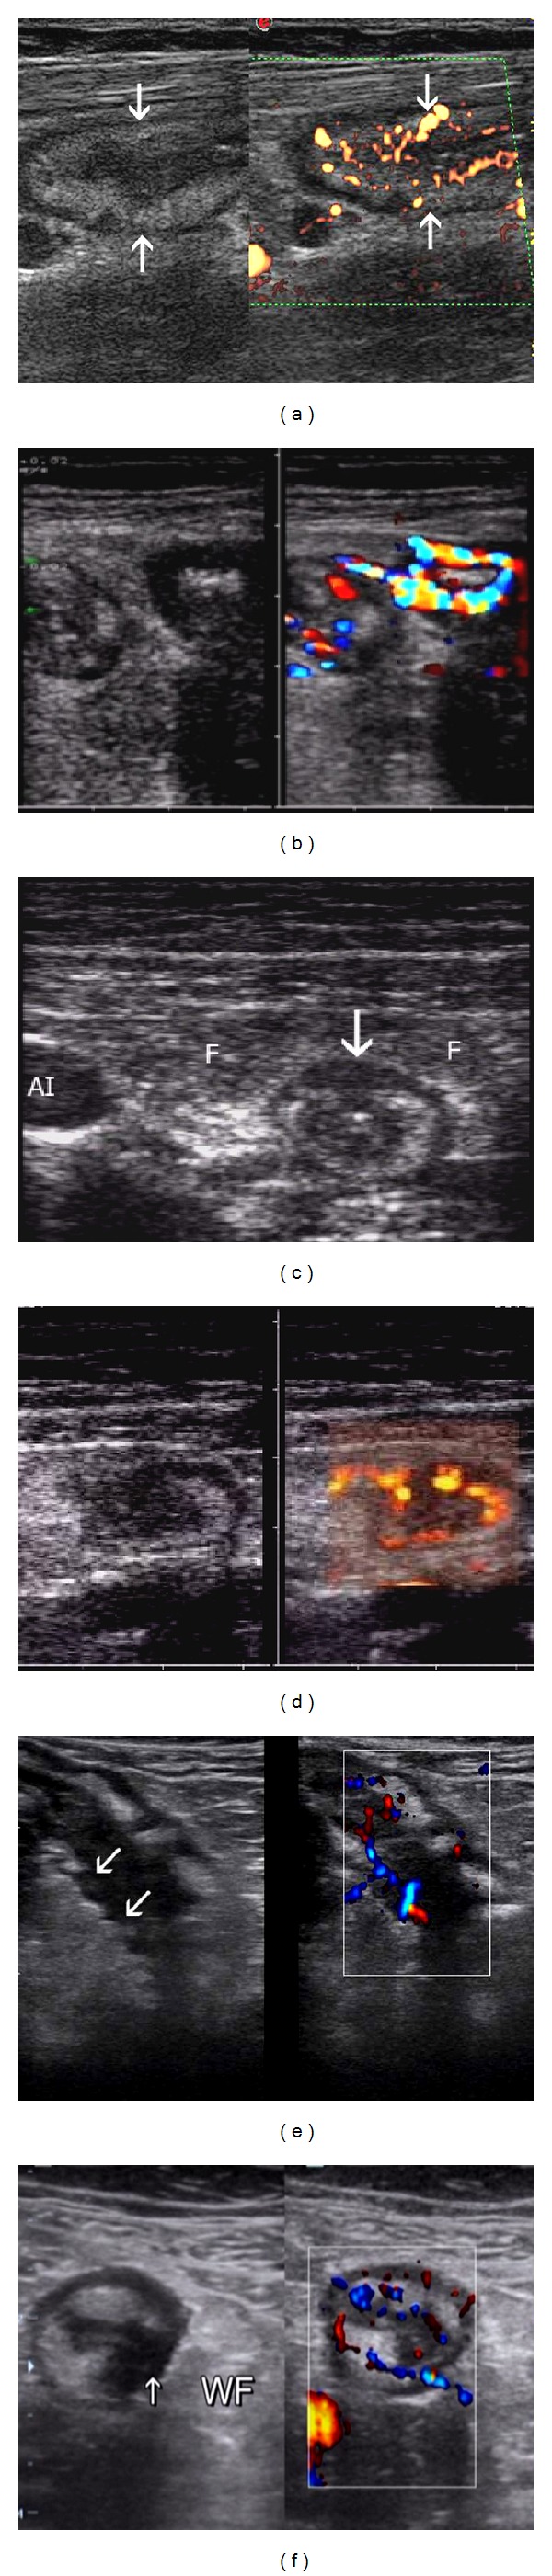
TUS in Crohn's disease. (a) Transversal view of thickened terminal ileum with preserved stratification and intramural hyper-vascularisation—*High resolution probe. *(b) Transversal section of two ileal bowel loops—proximal (left) with segmentally impaired stratification, distal with complete absence of stratification with hypoechogenic wall. The right half of picture shows intramural hyper-vascularisation especially in proximal loop, indicating active inflammation—*High resolution probe*. (c) Transversal view of terminal ileum with hypoechogenic bridge through echogenic submucosa between lumen and outer surface of the wall indicating transmural ulcer (arrow) and thickened inflamed “wrapping” fat (F)—*High resolution probe*. (d) Blind fistula wrapped by inflamed fat. Increased intramural vascularisation in color-Power Doppler (CFD)—*High resolution probe.* (e) Segmental absence of echogenic submucosa indicates longitudinal ulcer of terminal ileum (arrows) in longitudinal and (f) transversal view in a Crohn's ileitis (WF-inflamed fat)-FDsign—*High resolution probe. *
